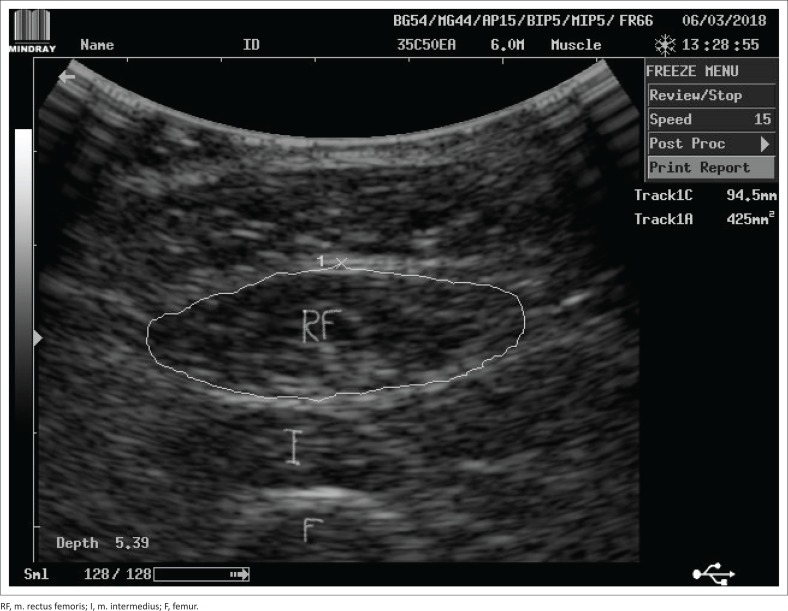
Example of measurement of cross-sectional area of the rectus femoris muscle of a healthy individual using on-screen callipers of the ultrasound diagnostic imaging system.
